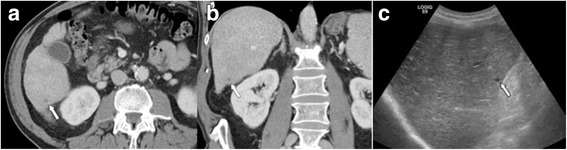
LR-2. Venous phase MDCT in the a axial and b coronal plane shows a small indistinct hypoattenuating observation in the right lobe (arrows). No enhancement was seen in other phases. Diagnosis of probable cyst (LR-2). c Ultrasound was performed, which confirmed the presence of a small subcapsular cyst (arrow)
